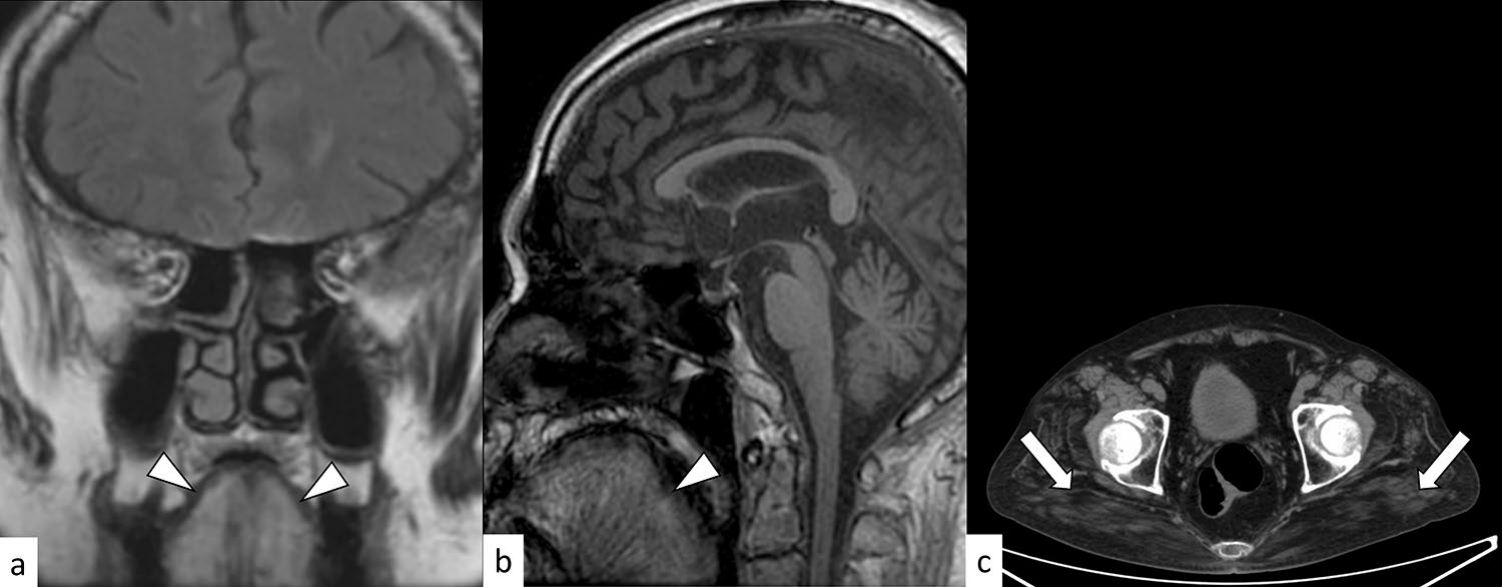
A 71-year-old man with SBMA. FLAIR coronal image a and T1-weighted sagittal image b show hyperintensity atrophic tongue muscles, suggestive of fat infiltration (arrowheads). Non-enhanced axial CT image shows fat infiltration in the bilateral gluteal muscles (c, arrows)
